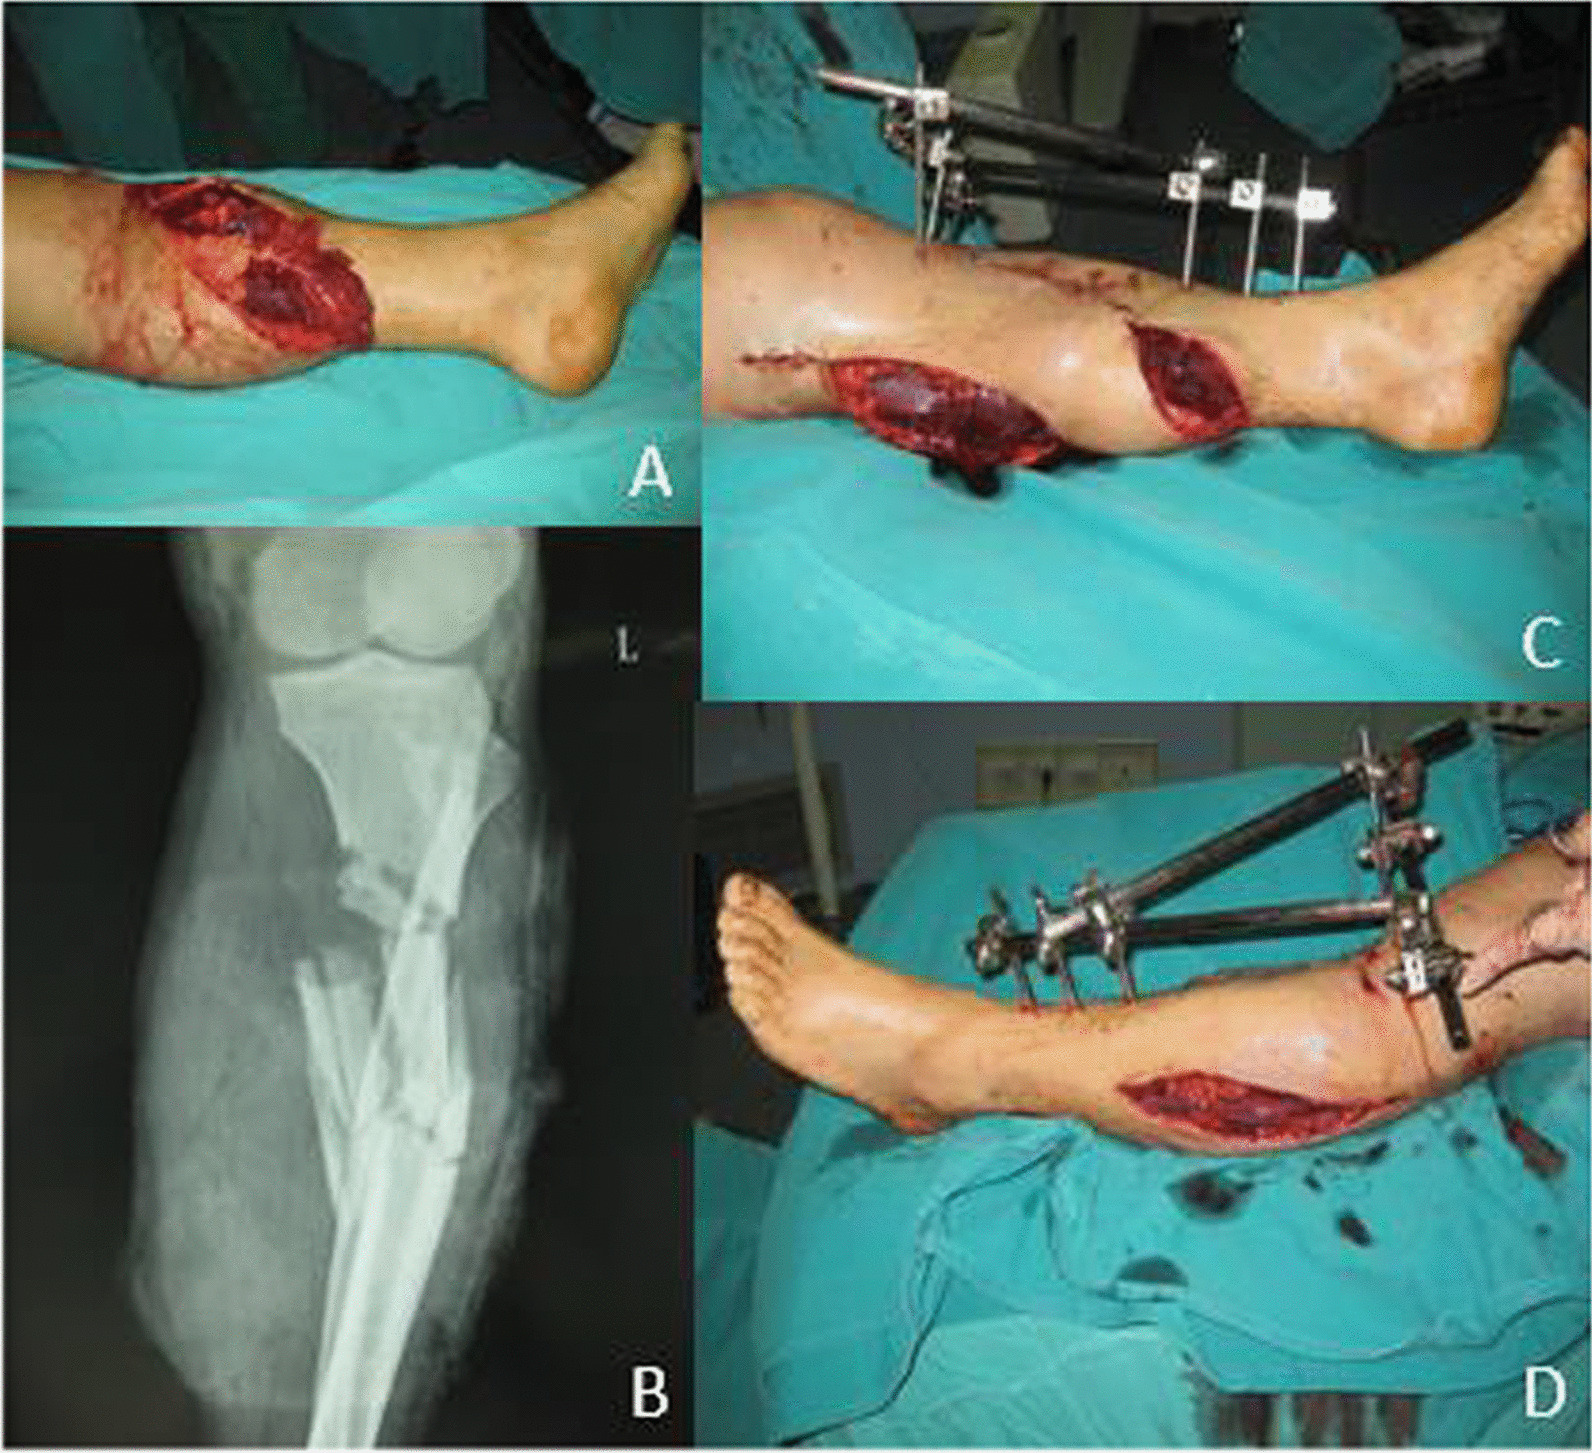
An example of the classification and the application of external fixation. A The patient was classified as type IIIc by Gustilo classification. B The preoperative radiograph showed an open comminuted fracture of the left tibia and fibula. C, D External fixation was used
